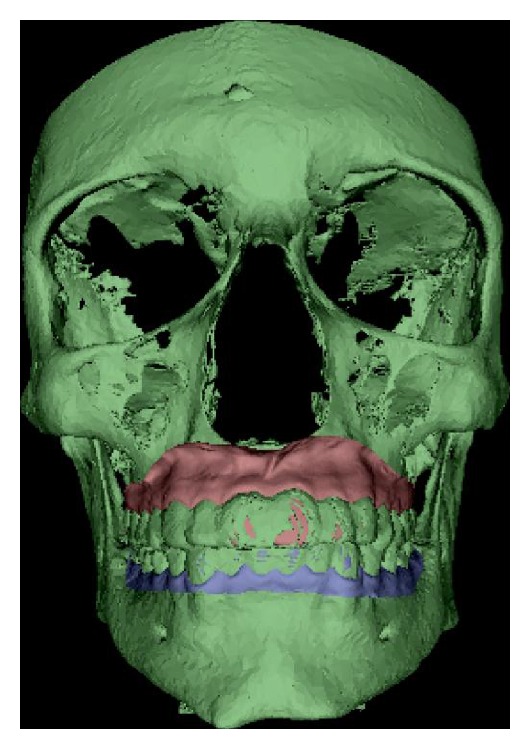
Superimposition of the 3D-scanned models with the CBCT to find the threshold value.
